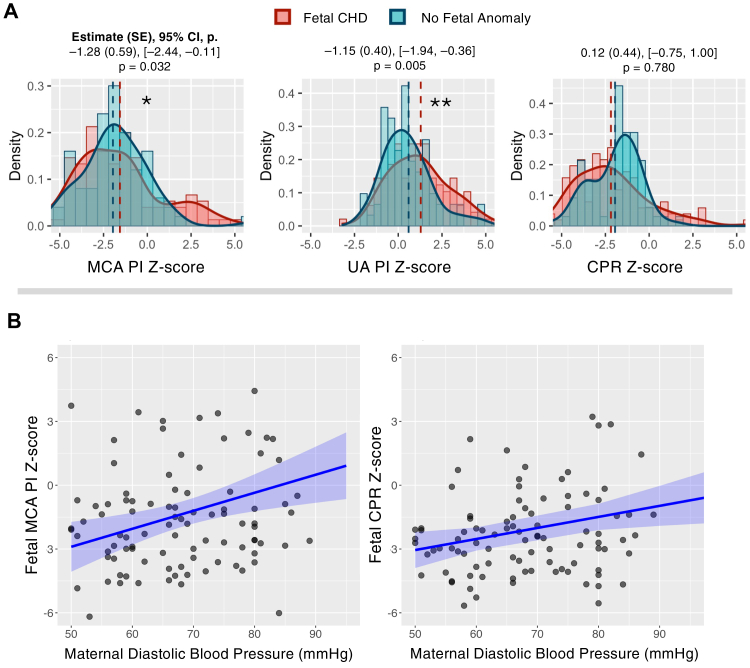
Maternal diastolic BP is associated with cerebral blood flow in foetuses with CHD. (A) Comparing the distribution of Z-scores of Foetal Doppler measurements: Pulsatility Index (PI) Z-scores in foetuses with CHD (red) vs. no foetal anomaly (blue), in the Middle Cerebral Artery (MCA), Umbilical Artery (UA) and Cerebroplacental Ratio (CPR). Statistics reflect the group difference (CHD – No Foetal Anomaly) in z-score for each Doppler measure, adjusted for foetal sex, GA, single ventricle physiology, maternal age, maternal BMI, foetal head circumference and estimated foetal weight. (B) (Left) The relationship between maternal diastolic blood pressure (mmHg) and foetal MCA PI. (Right) The relationship between maternal diastolic blood pressure (mmHg) and foetal CPR ratio. Each point represents an individual observation, and the solid blue line shows the adjusted effect of diastolic BP on MCA PI from a multivariate linear regression model (holding continuous covariates at their mean; GA, single ventricle physiology, maternal age, maternal BMI, foetal head circumference) with the shaded blue area representing the 95% confidence interval.
